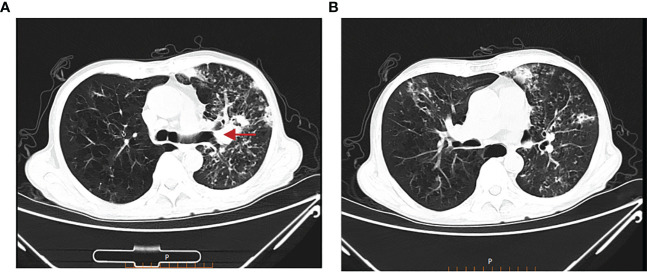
Chest CT on 22 May 2022. (A) The lung cancer in the upper lobe of the left lung was less extensive than before. (B) Multiple small nodules and small patchy high-density shadows in both lungs were more extensive than before.
